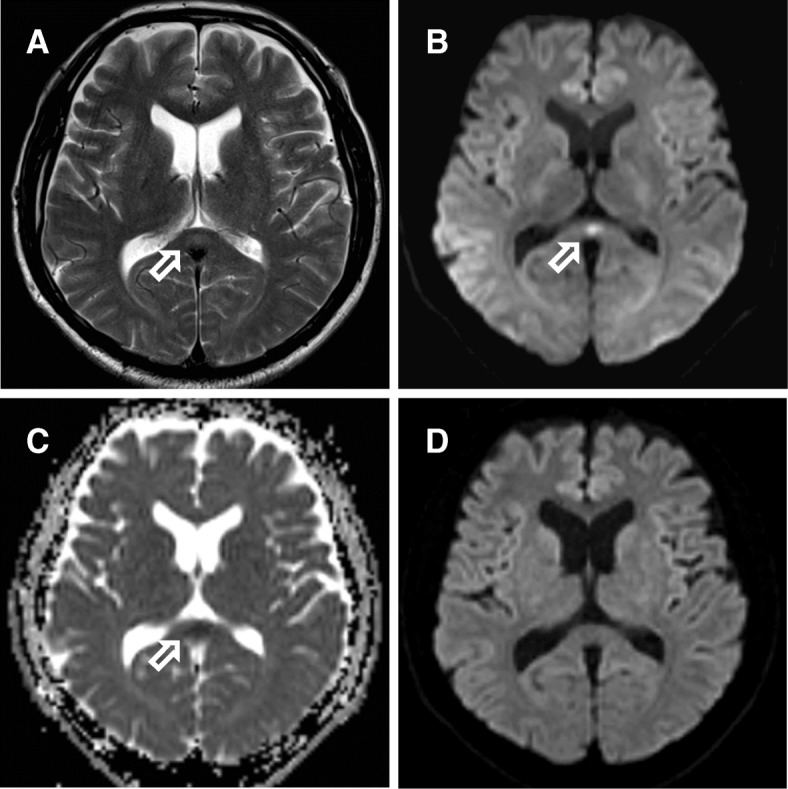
a–c Brain magnetic resonance images on day 3 of illness. a The T2-weighted image and b the diffusion-weighted image show an oval-shaped high-signal lesion in the splenium of the corpus callosum (arrow). c The apparent diffusion coefficient is decreased. d Brain magnetic resonance diffusion-weighted image on day 16 shows complete resolution of the lesion
